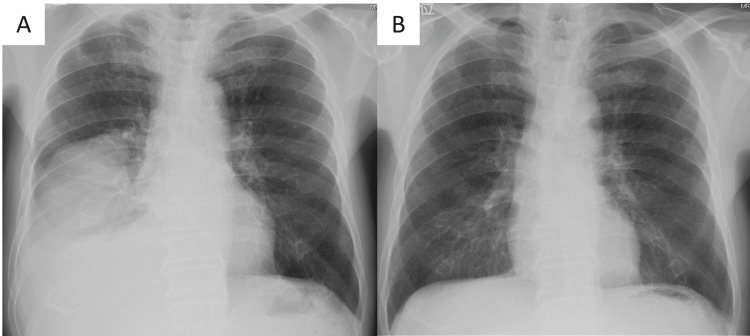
Chest X-ray findings of chest of first admission and one year ago Chest X-ray showed huge mass in the right lower lung field (A), which had not been detected one year ago (B).
